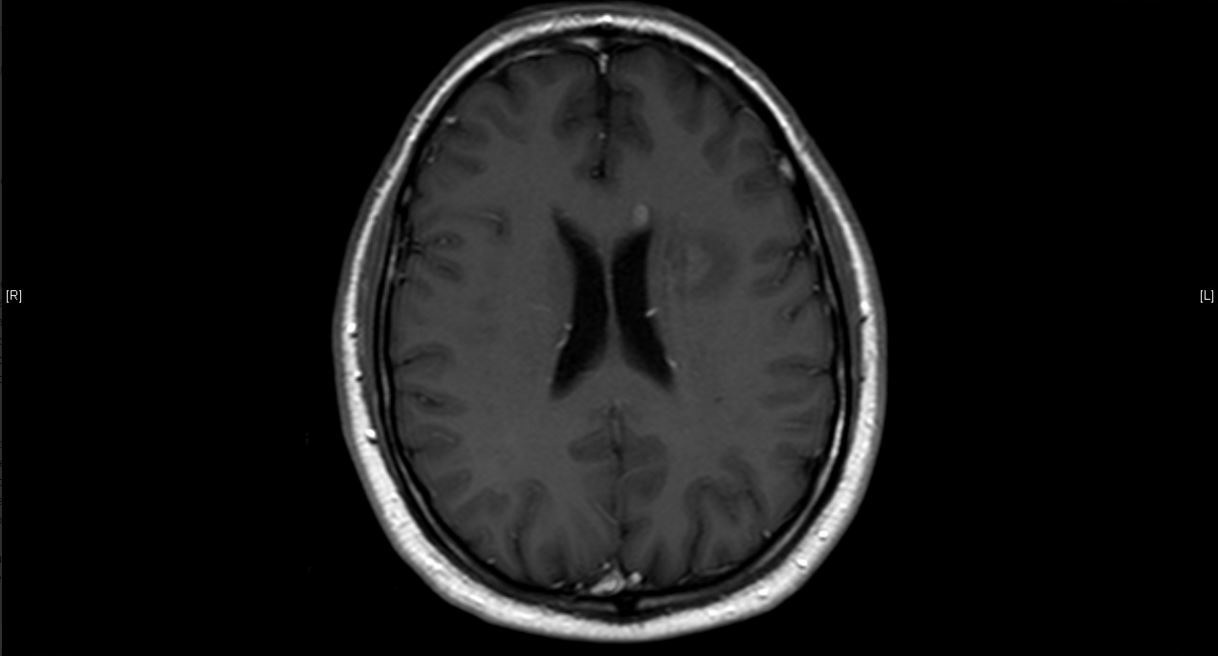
T1 with contrast MRI image showing a periventricular enhancing lesion anteriorly in the left side of the genu of the corpus callosum. The larger periventricular open ring cystic lesion is also noted in the same image.
